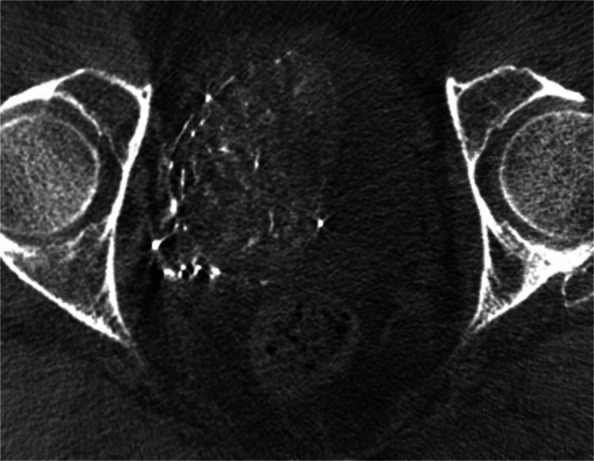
CBCT from right prostate artery injection
